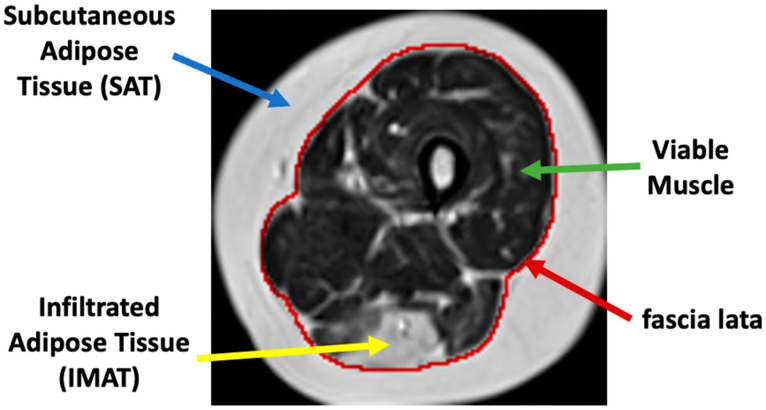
Axial MR image of the thigh. Red: fascia lata boundary; blue, green, and yellow arrows mark the subcutaneous fat, a region of viable muscle, and IMAT pixels.
